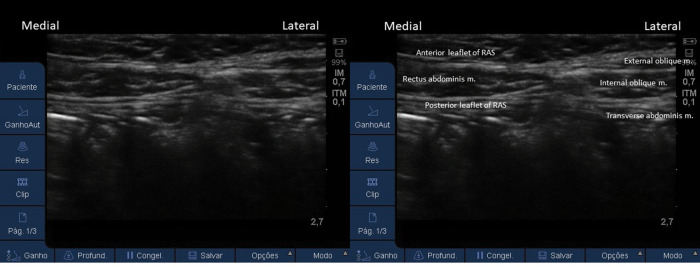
Ultrasound image of the RA muscle enveloped by its sheath and the plane muscles, laterally.
